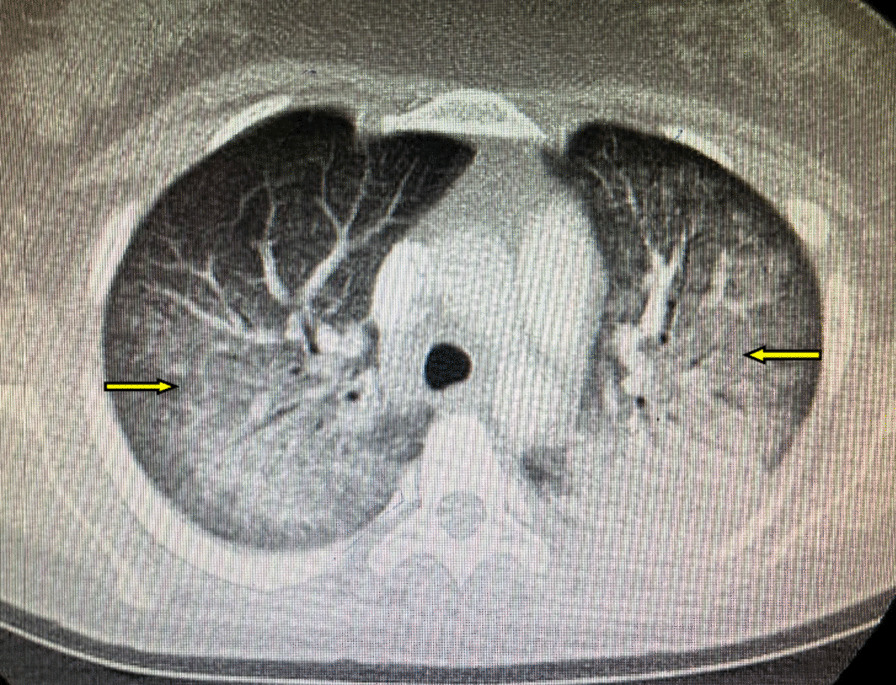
Chest CT scan demonstrating infiltrations of both lungs (arrows)
